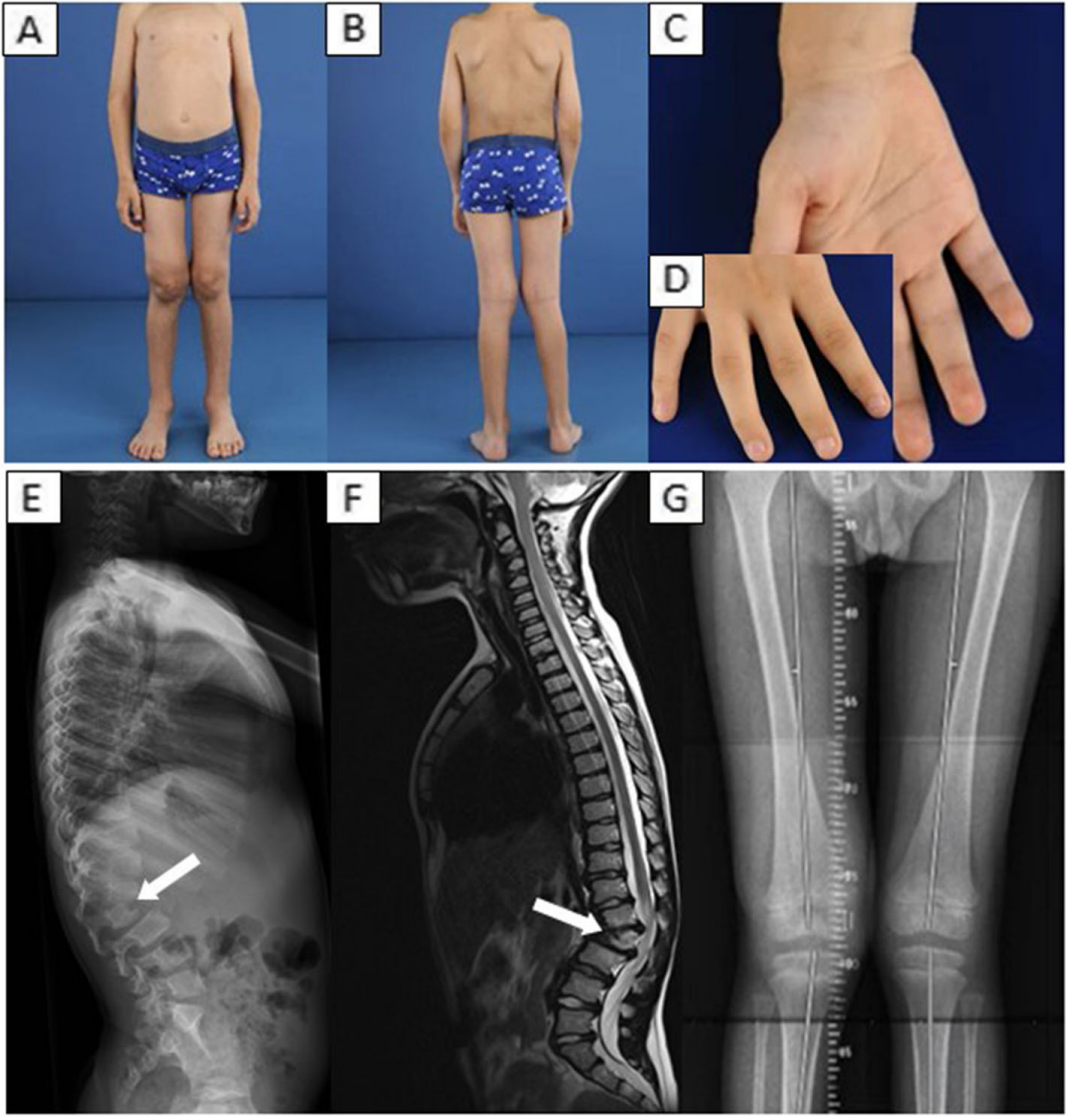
Clinical photographs (age: 8-years-old) revealing genu valgum, hydrops located in the right knee joint (A and B), ulnar deviation (C) and mild contractures of the 2nd, 3rd and 4th proximal interphalangeal joints (D). X-rays and MRI showing thoracolumbar kyphosis based on L2 hypoplasia (E and F) and valgus malalignment in both knees (G)
